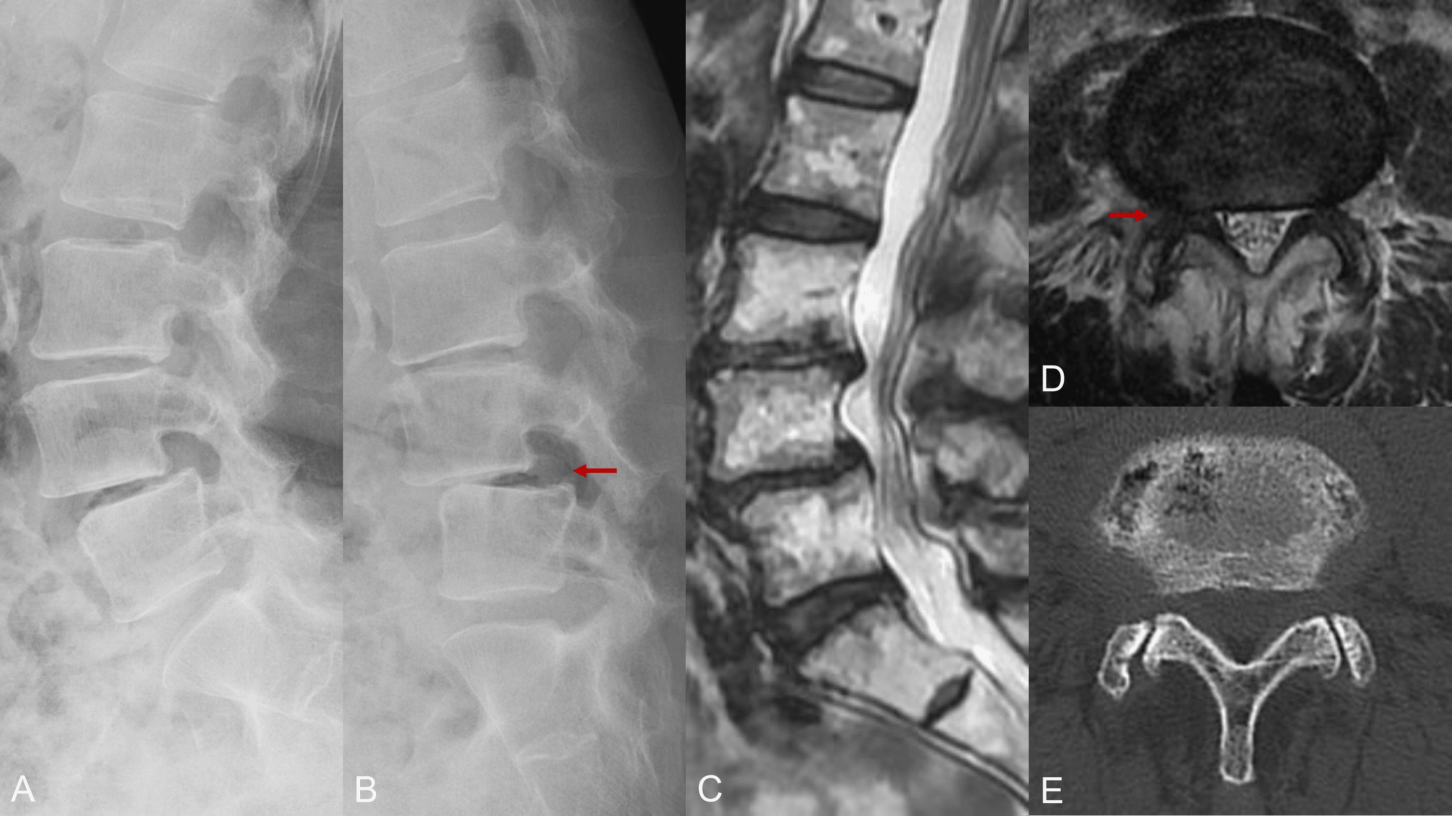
Preoperative imaging (A, B) Plain X-ray showed L4 spondylolisthesis with instability (arrow); (C, D) Sagittal and axial MRI showed lumbar canal stenosis at the L4/5 level (arrow); (E) Axial CT scans at the L4/5 level
